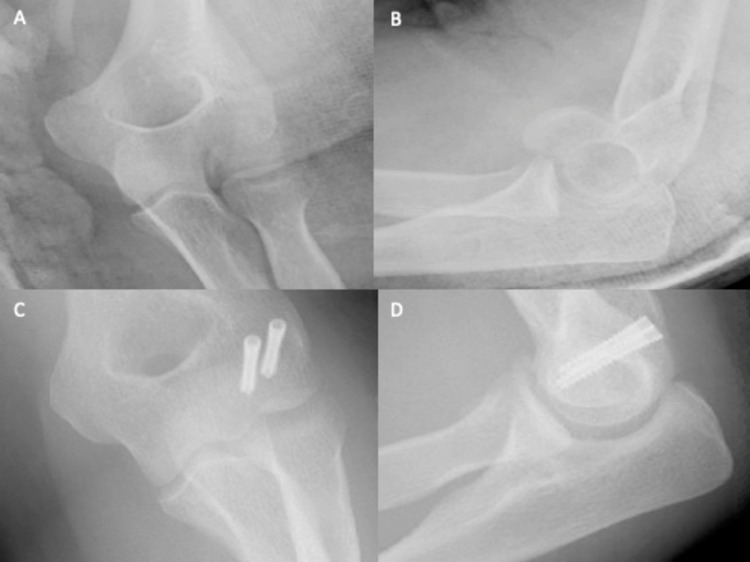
Plain elbow X-rays of case 3. Figure 4A, 4B shows anteroposterior and lateral views, respectively, of a type II capitellum fracture. Figure 4C, 4D shows anteroposterior and lateral views, respectively, of the elbow after ORIF. ORIF: open reduction and internal fixation
